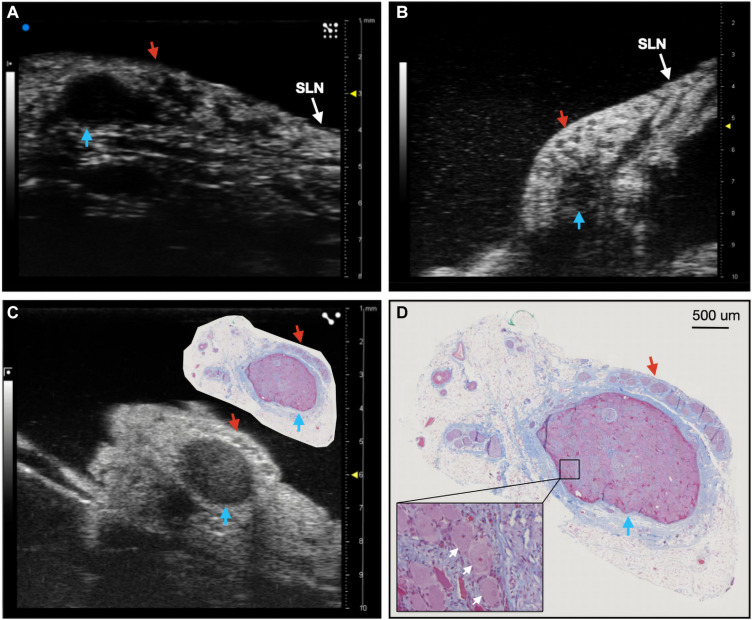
Ultrasound of the nodose ganglia in two live, and one cadaver subject (*n* = 3). Blue arrows indicate the hypoechoic pseudo-unipolar cell region of the nodose ganglia, red arrows indicate the motor efferent region fascicles. (A,B) Ultrasound of the nodose ganglia and superior laryngeal nerve (SLN) *in vivo.*
